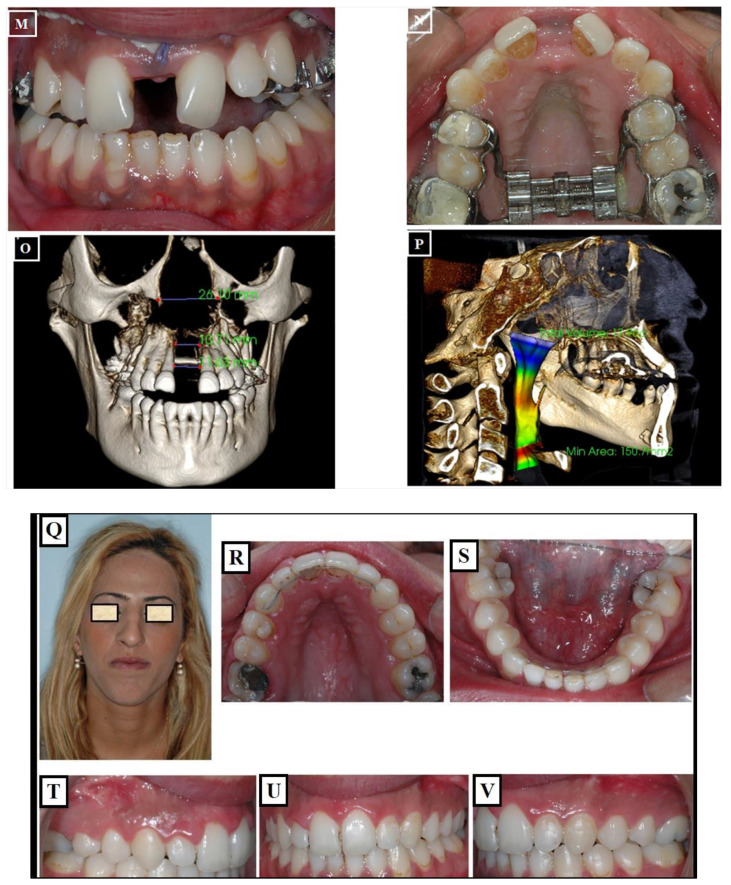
Expansion of the maxilla through Surgically Assisted Rapid Palatal Expansion (SARPE). Situation before treatment: narrow face and narrow nose (A), extremely narrow upper jaw (blue arrows 21mm, 33 mm) (B) compared to the lower jaw (blue arrows 31mm, 43 mm) (C), skeletal and dentoalveolar crossbite on the right and left (D–F). Surgical assistance for maxillary expansion. Surgical separation of the bones on the maxilla was partially performed at the level of Lefort I (G–L). Maxillary expansion with the screw (M,N). Cone-beam computed tomography (CBCT) after expansion shows the separation of Maxillary parts, as part of an examination, the amount of expansion at the bone was measured compared to the screw opening (O) and the airway the colored area represents the measured airways before and after expansion (P). Improvement in breathing and breathing disorders is expected in such patients. Condition after treatment, changes in facial width (Q), changes in jaw widths (R,S) with occlusion correction (T–V).
